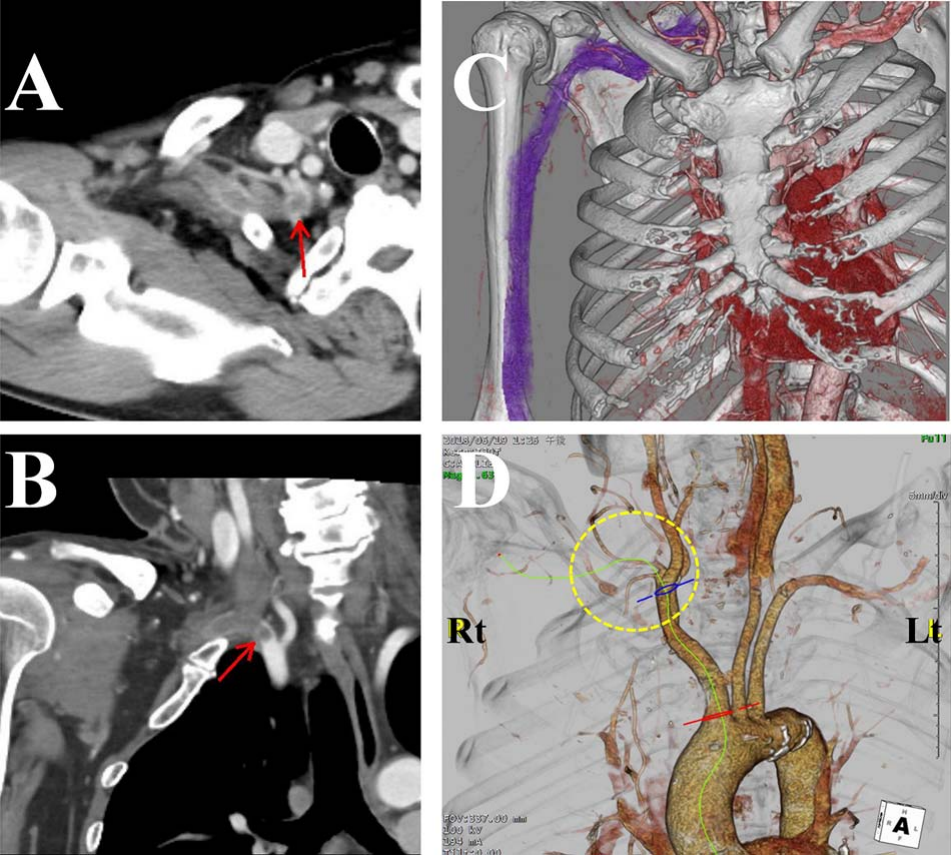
Contrast-enhanced computed tomography (CT) images of the thorax. (A) Axial view. (B) Coronal view. (C, D) Three-dimensional CT images. The subclavian artery (circle) was occluded due to a thrombus (arrow) at the level of the dysplasia of ribs.
